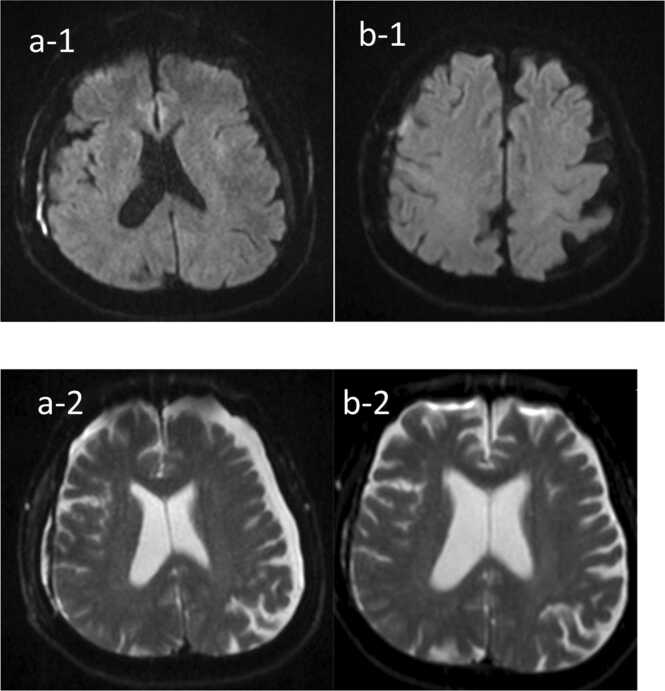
The brain MRI on 31th (a-1, a-2) day and 2weeks after discharge (b-1, b-2). [a-1], [a-2] The abscess on the surface of the right frontal lobe shows signs of shrinking. However, a new abscess has appeared in the right lateral subdural space of the frontal lobe. [b-1], [b-2] The abscess on the surface of the right frontal lobe shows further shrinkage. The abscess in the right lateral subdural space of the frontal lobe, newly observed in the previous MRI ([a-1], [a-2]), has also decreased in size.
